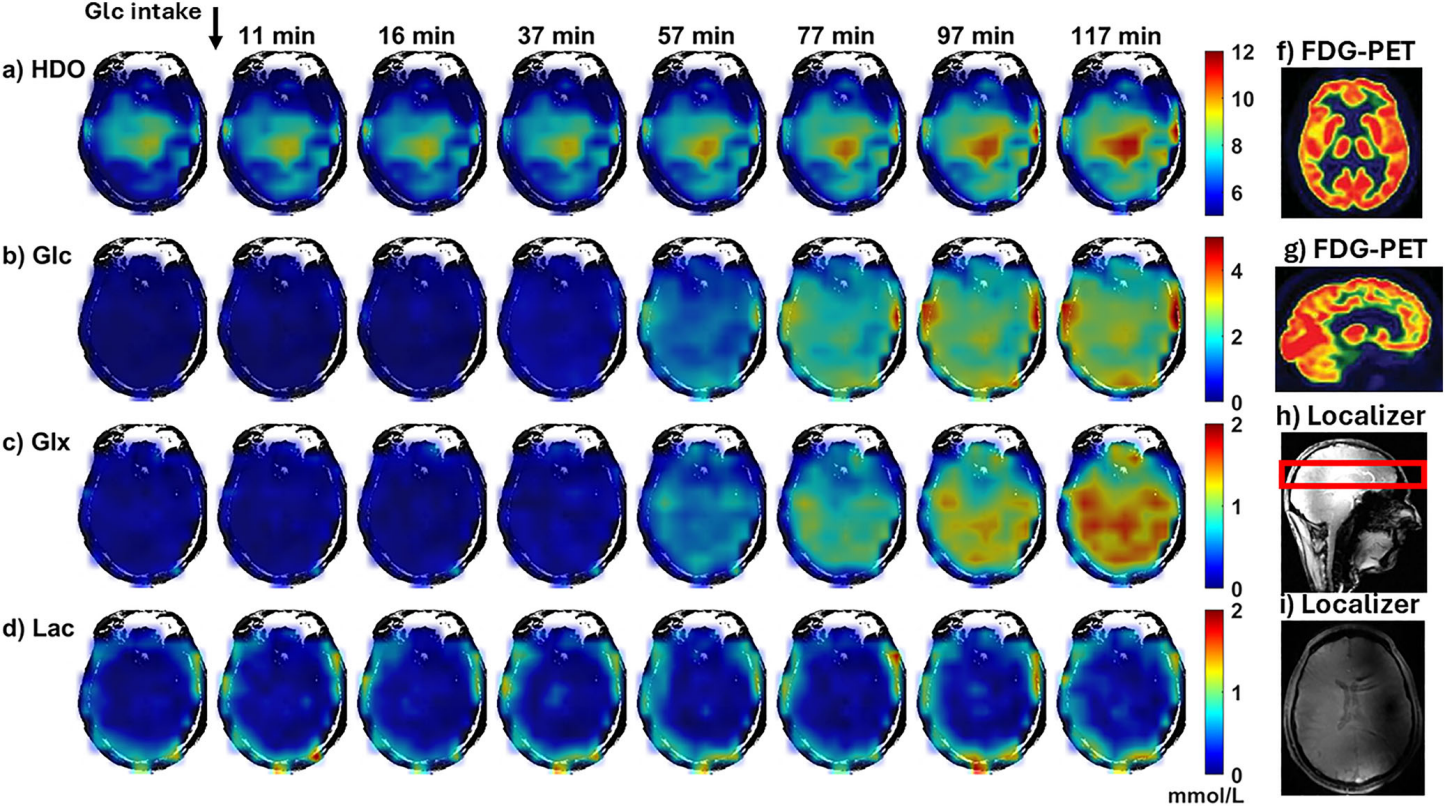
Evolution of metabolite maps evolution at baseline and following oral [6,6‐^2^H_2_]‐glucose administration to a healthy volunteer. HDO, Glc, Glx and Lac maps are shown in (a), (b), (c), and (d), respectively after application of the whole brain mask #1. Baseline DMI scans were acquired only in healthy volunteers. This was only done in healthy volunteers so that the patients didn't have to enter the magnet twice. Transaxial (f) and sagittal (g) ^18^F‐FDG PET images of a normal subject are shown, reproduced from Berti et al., PET Clinics, 2014 [72], Figure 2, with permission, alongside a sagittal (h) and transverse (i) GRE image for this volunteer.
